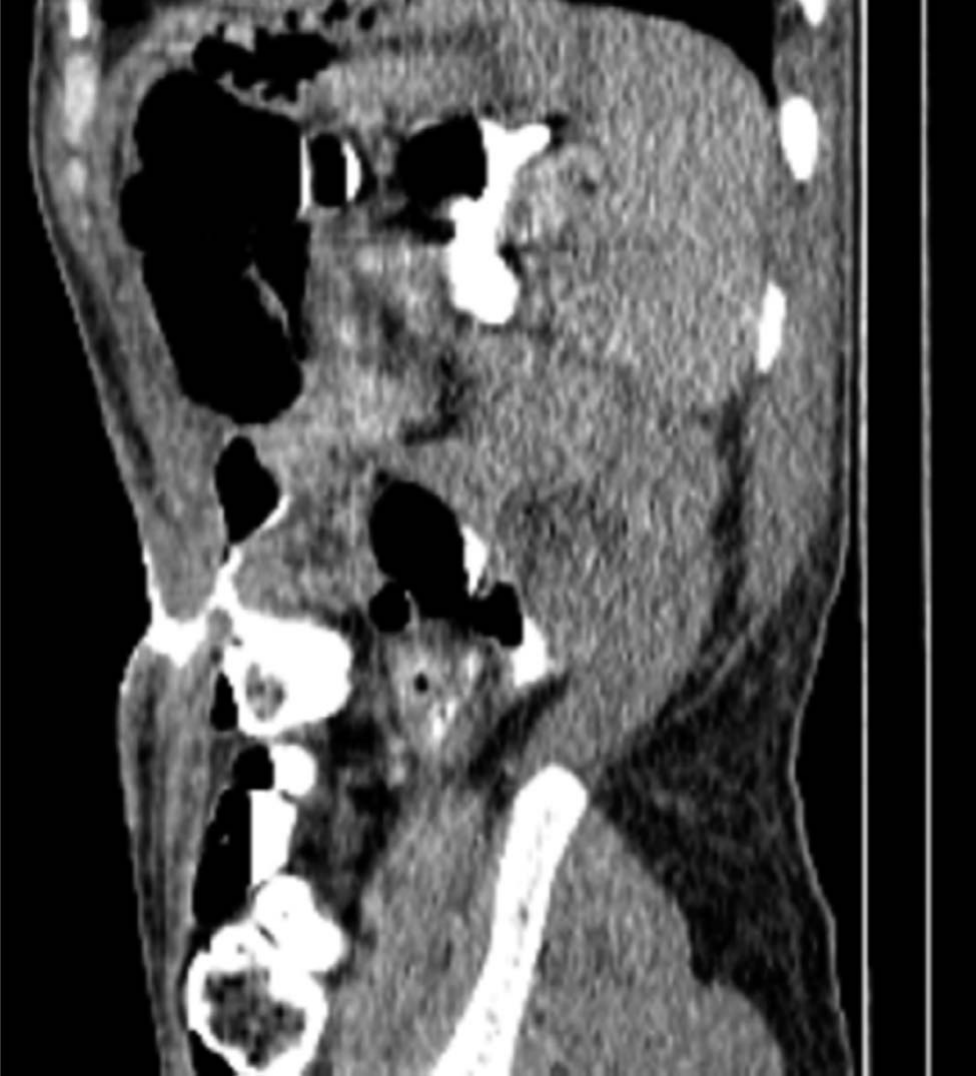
Oral contrast computed tomography showing the fistula tract.
